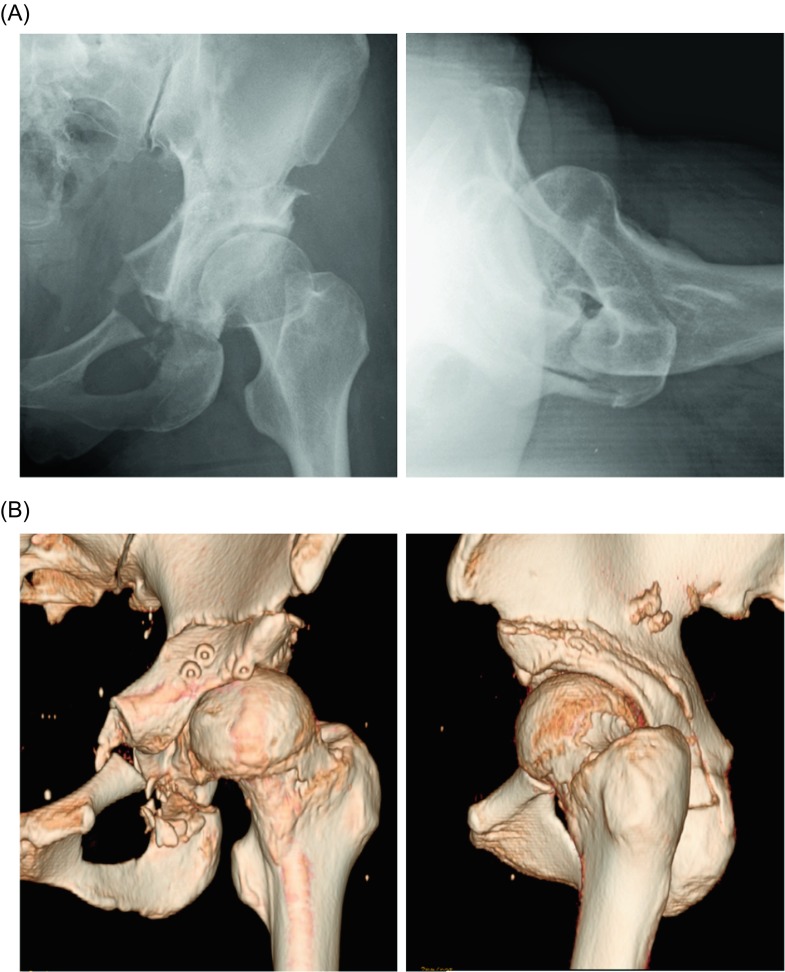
Pre-operative radiograph showing slight osteoarthritis due to hip dysplasia (A); bump on the femoral head-neck junction, with an alpha angle of 75° (B); pre-operative three-dimensional reconstruction of the computed tomography (CT) image (C).
